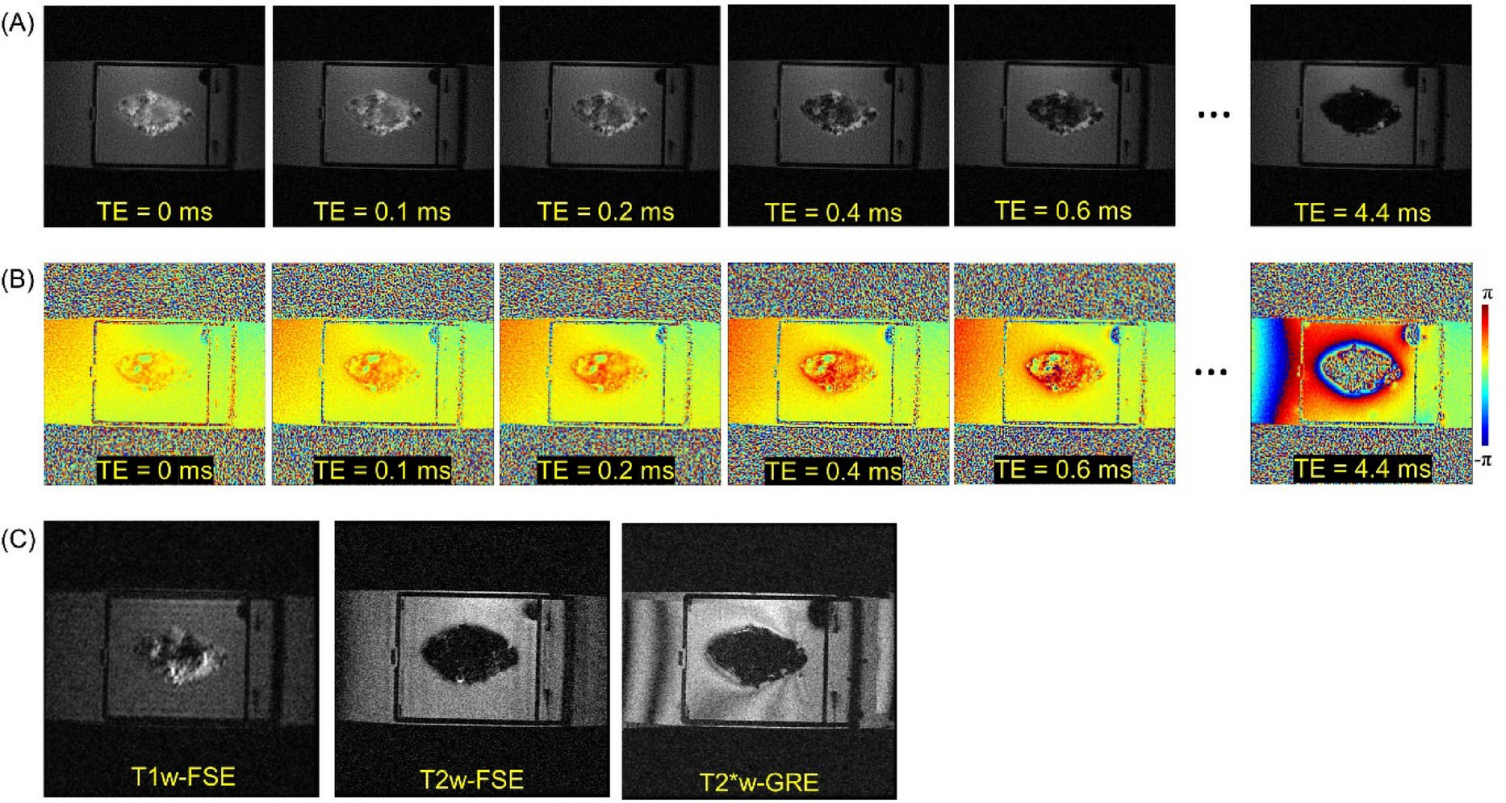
UTE-QSM and the corresponding clinical MRI images. (A) The magnitude and (B) the phase of UTE-Cones images at different TEs, and (C) the corresponding clinical T1w-FSE, T2w-FSE, and T2*w-GRE images.
